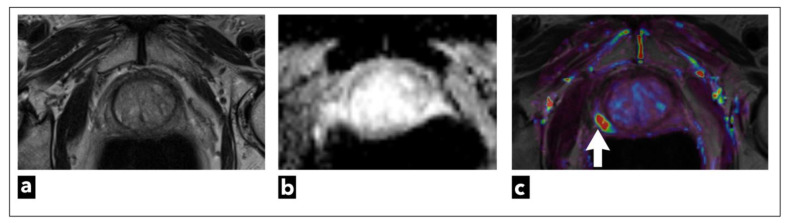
mpMRI shows a right peripheral zone prostate lesion (white arrow) in a 72-year-old male patient, which was confirmed to be cancer on biopsy; Gleason score 3 + 3 = 6. The images present (a) T2W as a lightly hypointense focal lesion, obscured on (b) ADC map due to artifacts, and the most noticeable on (c) DCE-MRI, which demonstrates early contrast enhancement on the pharmacokinetic quantitative colour-coded parametric map for K_trans_.
